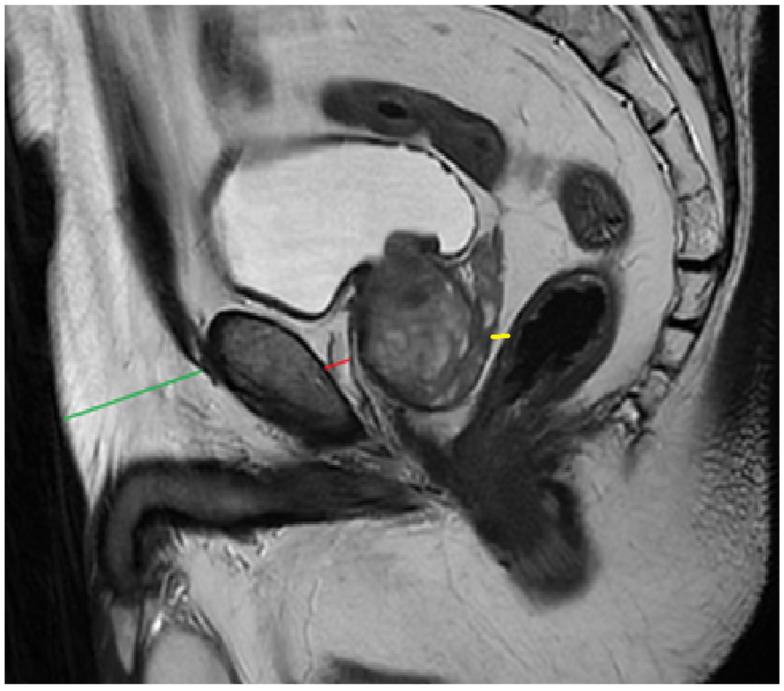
Measurement of PPAT (red line), posterior PPAT (yellow line), and SATT (green line) on a midsagittal view, T2 sequence, on a prostate MRI categorized as PI-RADS 4, showing a heterogeneous prostate gland that indents and protrudes into the urinary bladder.
